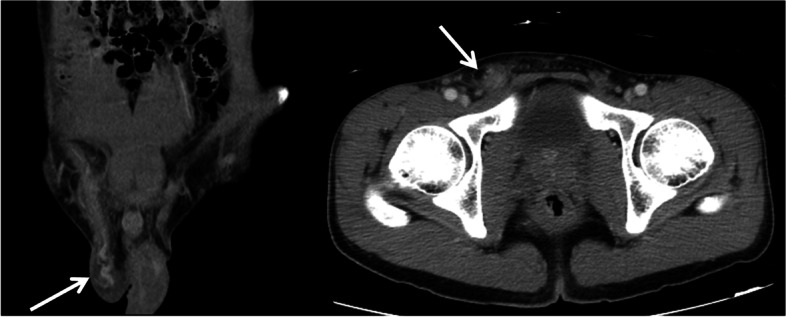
Contrast-enhanced CT. The coronal view shows multiple continuous vessels extending from the abdomen into the right inguinal area with surrounding fat stranding. Axial view shows right inguinal hernia. The white arrow highlights the equivocal lesion. Incarceration due to an ileal bowel loop with omental vessels cannot be excluded based on these images
